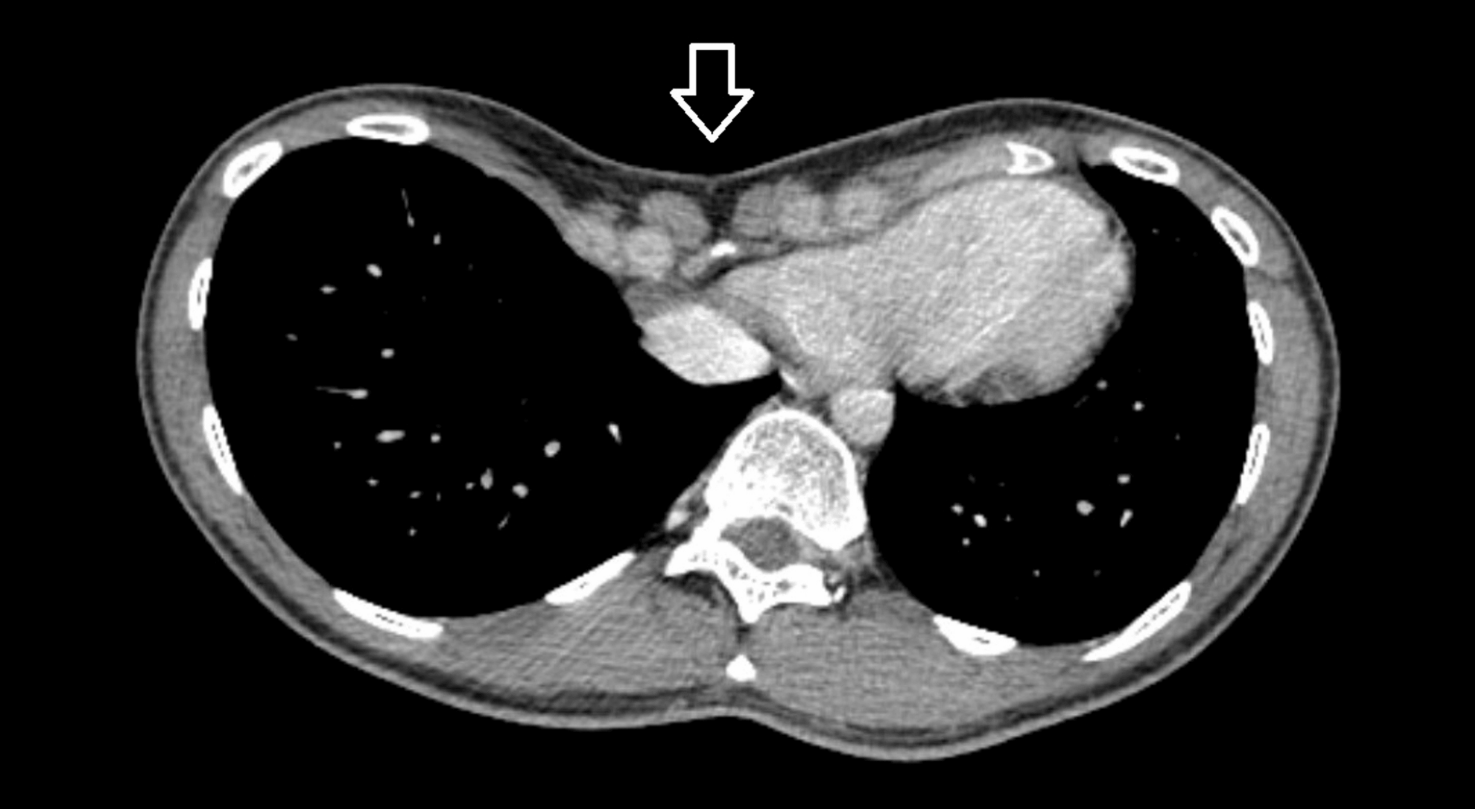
Computed tomography imaging with intravenous contrast Pectus excavatum (white arrow)
